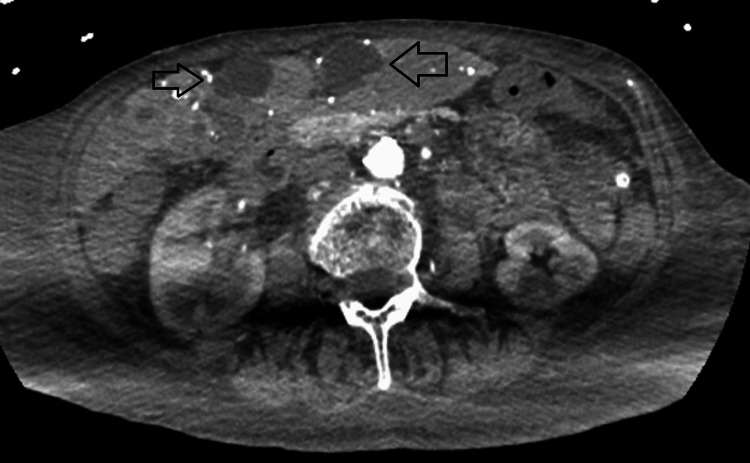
Computed tomography of the abdomen showing hepatic hypodense lesions (black arrows)
